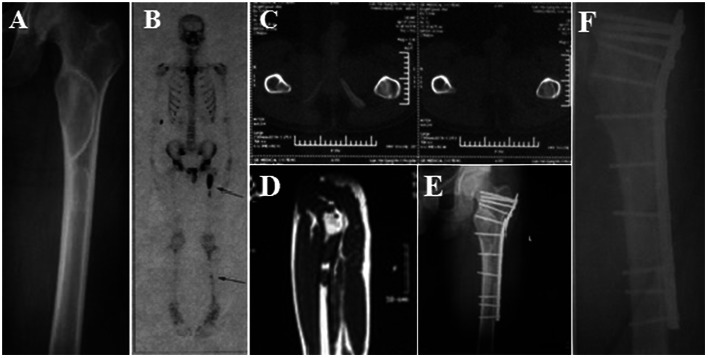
FD of the left proximal fumur of an 18-year-old man. (A) Anteroposterior preoperative radiograph; (B) preoperative radioisotope scanning; (C) pre-operative CT; (D) preoperative MRI; (E) anteroposterior postoperative radiograph; (F) anteroposterior radiograph shows bone union after 7 months of follow-up. FD, fibrous dysplasia.
